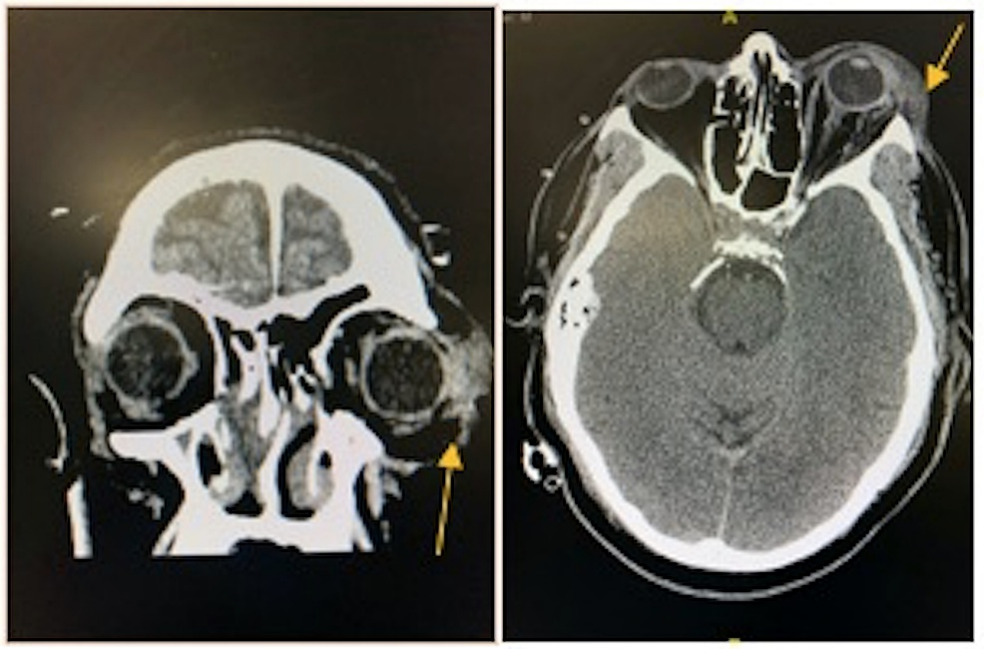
(A) CT brain coronal view (B) CT brain axial; yellow arrow pointing to soft tissue swelling left periorbital
